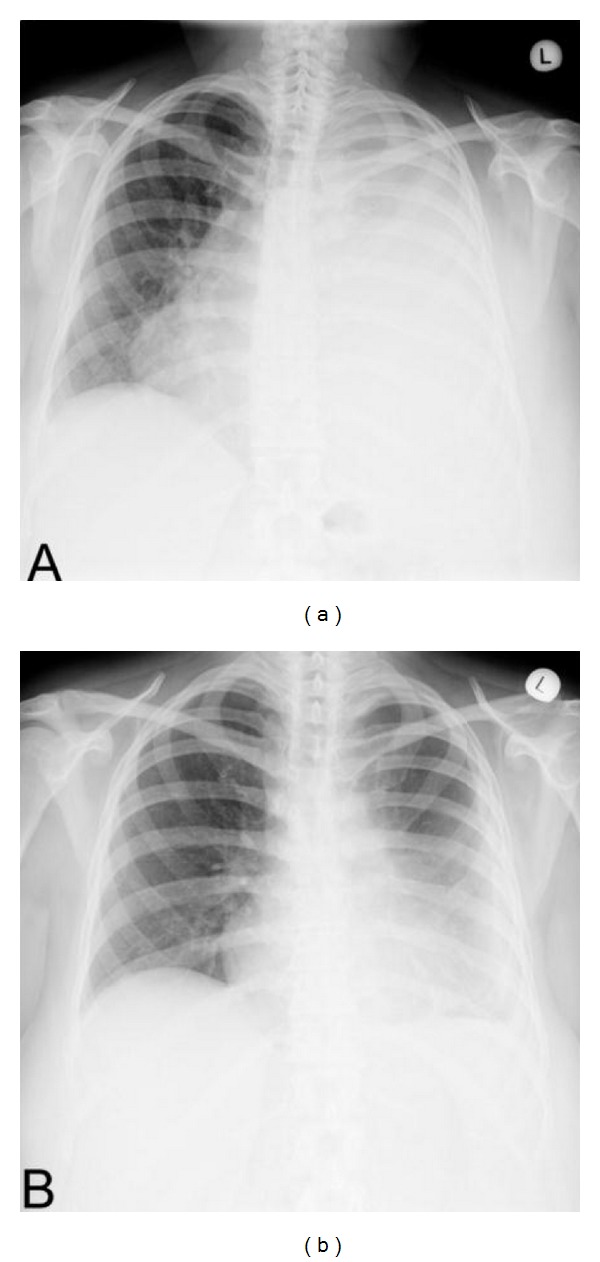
(a) Chest X-ray of patient showing massive left pleural effusion and (b) reduction of the pleural effusion after two doses of intrapleural bortezomib.
